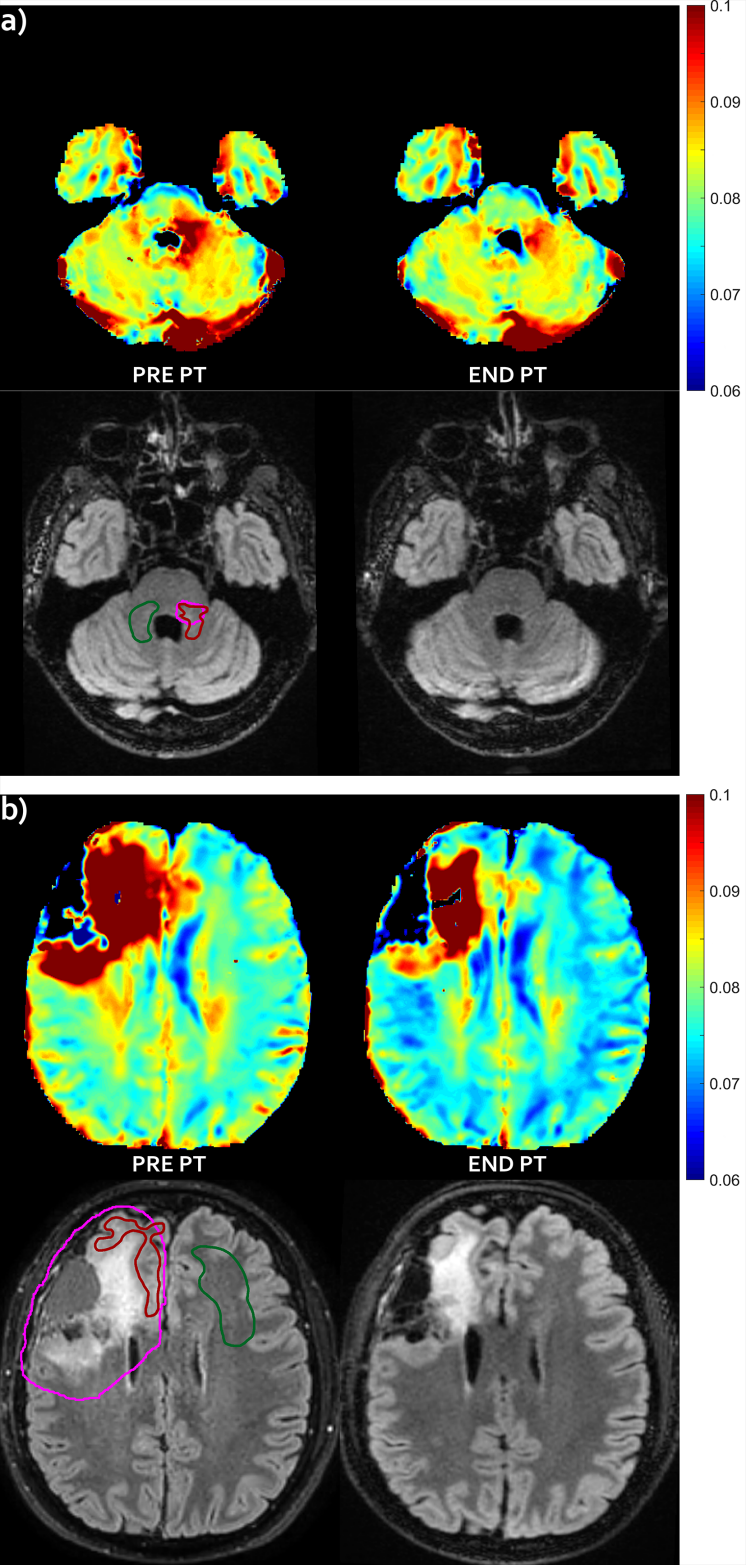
T2 decrease pattern—Evolution pattern observed in patient #1 (A) and #5 (B). The mismatch, i.e. abnormal T2 area that extended outside the hyper-intense FLAIR area before proton irradiation (left) markedly reduced at the end of the radiation treatment (right), with no appearance of successive FLAIR hyper-intensity in this region (not shown). For patient #1, no FLAIR abnormality was visible in panel (A), but it was visible at a different slice level (not shown). T2 intra/extracellular water maps (top) and FLAIR images (bottom) are shown. Colored bar is shown in seconds. On pre-PT FLAIR image the contours of the CTV (violet line), of the mismatch ROI (red line) and of the contralateral healthy ROI (green line) are shown.
